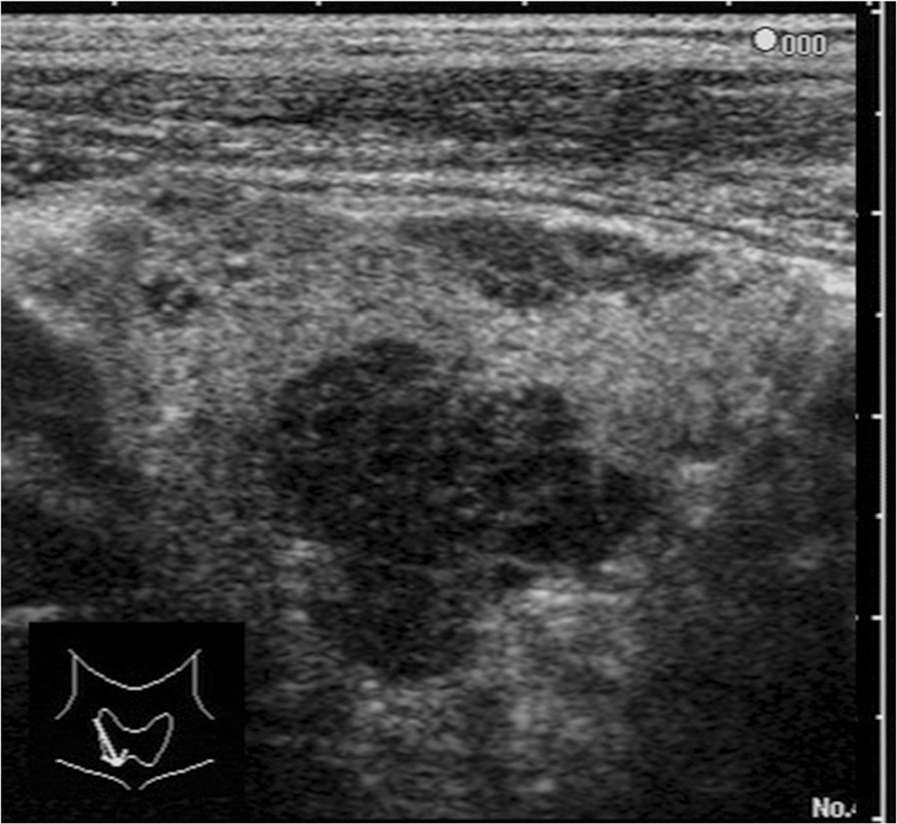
A representative ultrasonographic image. A well-demarcated lobular low-echoic lesion with diffuse short linear high-echoic spots inside in the thyroid was revealed by ultrasonography.
